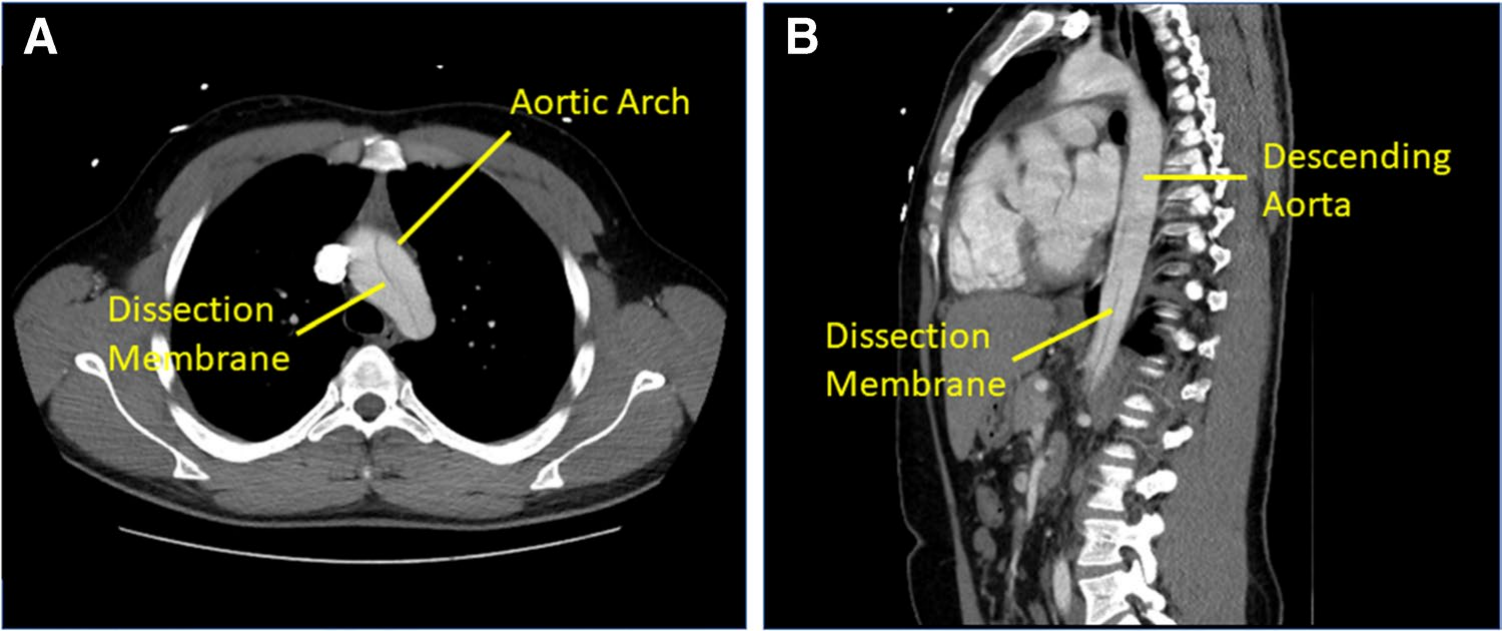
A Computed tomography (CT) demonstrated the aortic dissection and the aneurysm of the ascending aorta and the aortic arch. B The aortic dissection reached down the descending aorta to the level of the mesenteric artery
